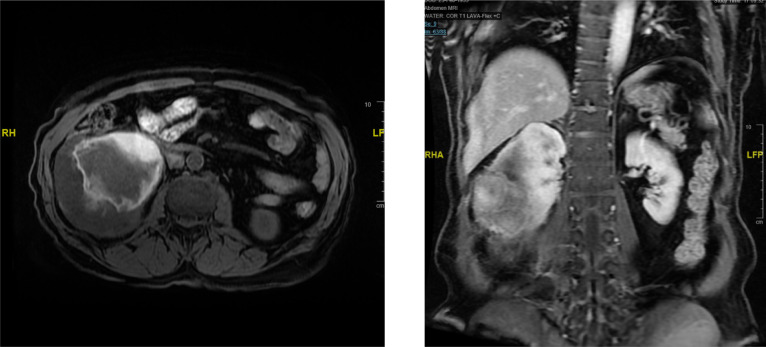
MRI of the abdomen shows there is a completely exophytic 3.8 × 8.5 cm soft tissue mass arising from the right inferolateral renal cortex and confined to the perinephric space with new angiogenesis without sinus fat or collecting system invasion, and no regional lymph nodes or renal vein invasion. No intra-abdominal metastasis.
